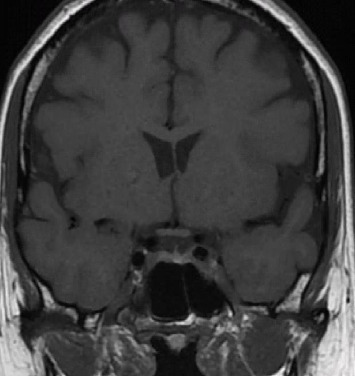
MRI sella—T1 coronal view showed small pituitary microadenoma.
